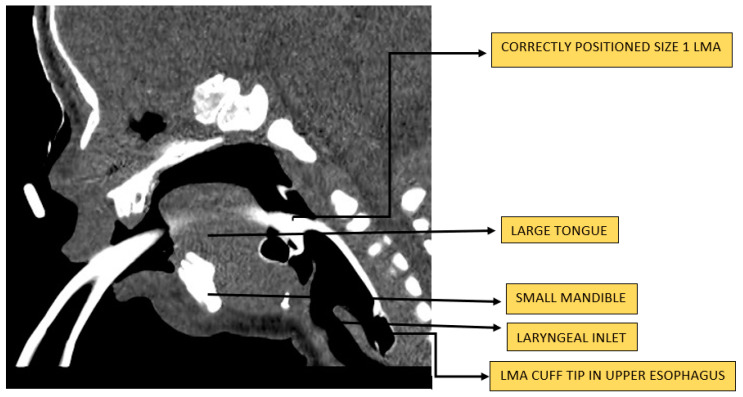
CT image of a size 1 LMA^®^ in situ in a neonate with severe micrognathia and congenital upper airway obstruction.
